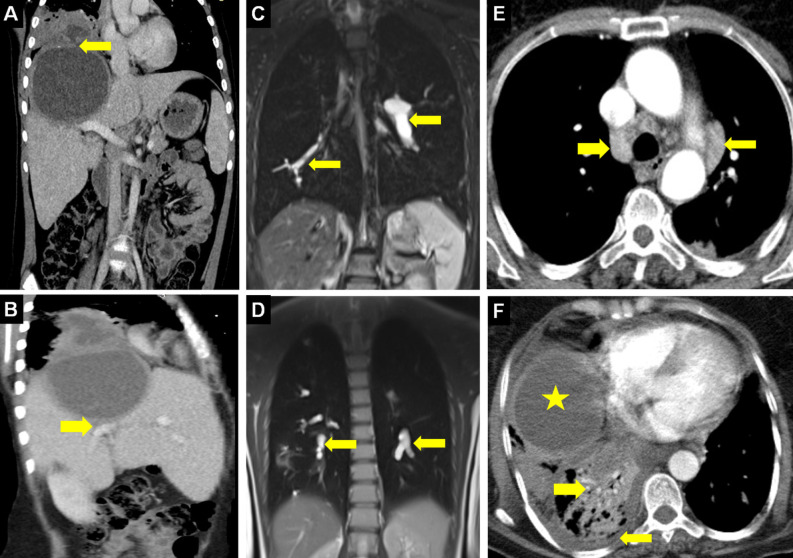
Coronal computed tomography (CT) (A) shows the fistula (arrow) of a hydatid cyst (HC) extending exophytically from the liver to the subdiaphragmatic area, to the diaphragm, and from there to the lung; and multiplanar reformatted sagittal section (B) shows a BBF with small connections to the intrahepatic bile ducts from inferior (arrow). Coronal T2-weighted magnetic resoance imaging (C, D) shows multiple hyperintense defects within pulmonary arteries (arrows) caused by intravascular hydatid content (hydatid embolism). Axial CT (E) shows mediastinal lymphadenopathies (arrows) due to ruptured and infected HC, and in a different case (F), an HC (asterisk) causing pleural effusion and consolidation (arrow) containing dilated air bronchograms (arrow) in the right lung is observed.
